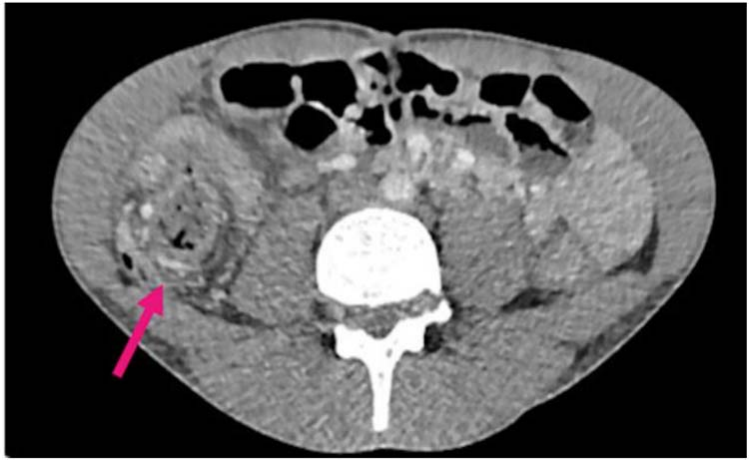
Contrast CT axial sections showing the enhanced thickened appendix (white) intussuscepts with fat stranding, into the ascending colon. Distal faecal loading also observed.
